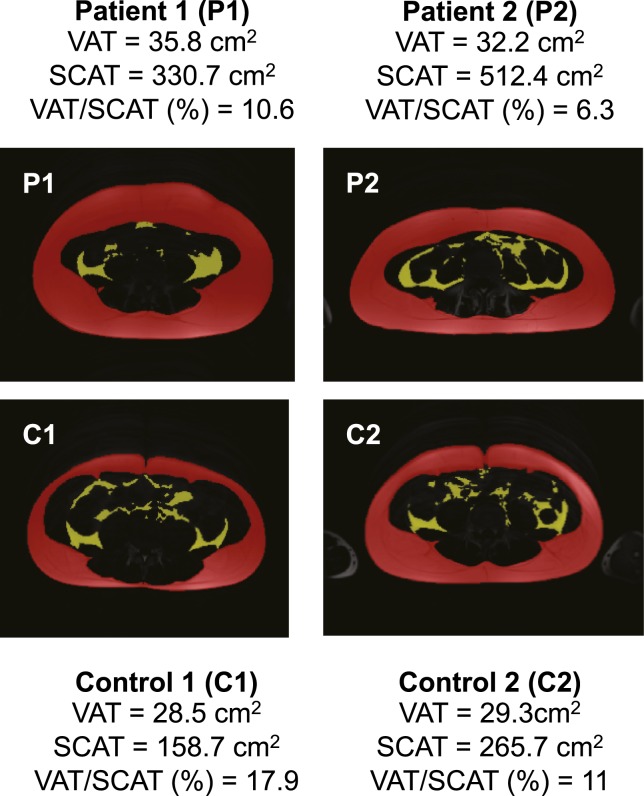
Quantification of visceral and subcutaneous adipose tissue. Representative abdominal fat distribution determined by magnetic resonance imaging at the L4 vertebral level is shown with pseudocolored adipose depots for P1, P2, and two healthy sex- and age-matched controls (C1, C2). Visceral adipose tissue (VAT) is indicated in yellow, and subcutaneous adipose tissue (SCAT) is indicated in red.
